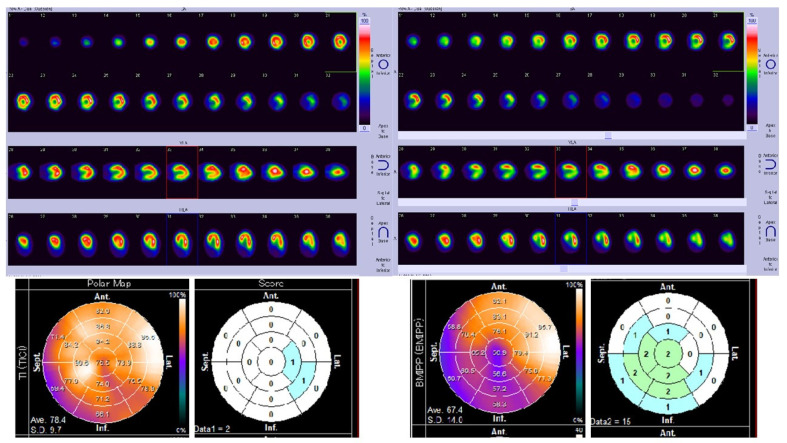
SPECT images, polar maps, and Heart Risk View-S scores of Case E whose LVEF decreased to 48.4%. Left top: SPECT images of ^201^Tl perfusion scintigraphy. Left bottom: polar map and scores of ^201^Tl. Right top: SPECT images of ^123^I-BMIPP metabolism scintigraphy. Right bottom: polar map and scores of ^123^I-BMIPP.
